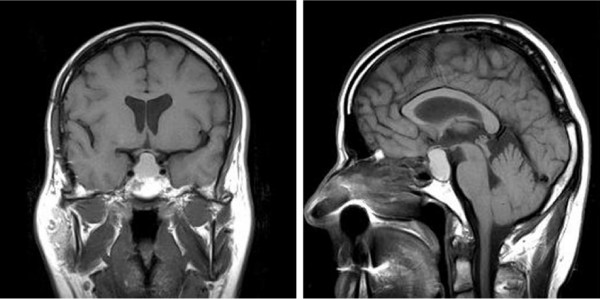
T1-weighted magnetic resonance images of cystic tumour of the described patient. Subsequently this was found to represent craniopharyngioma.
